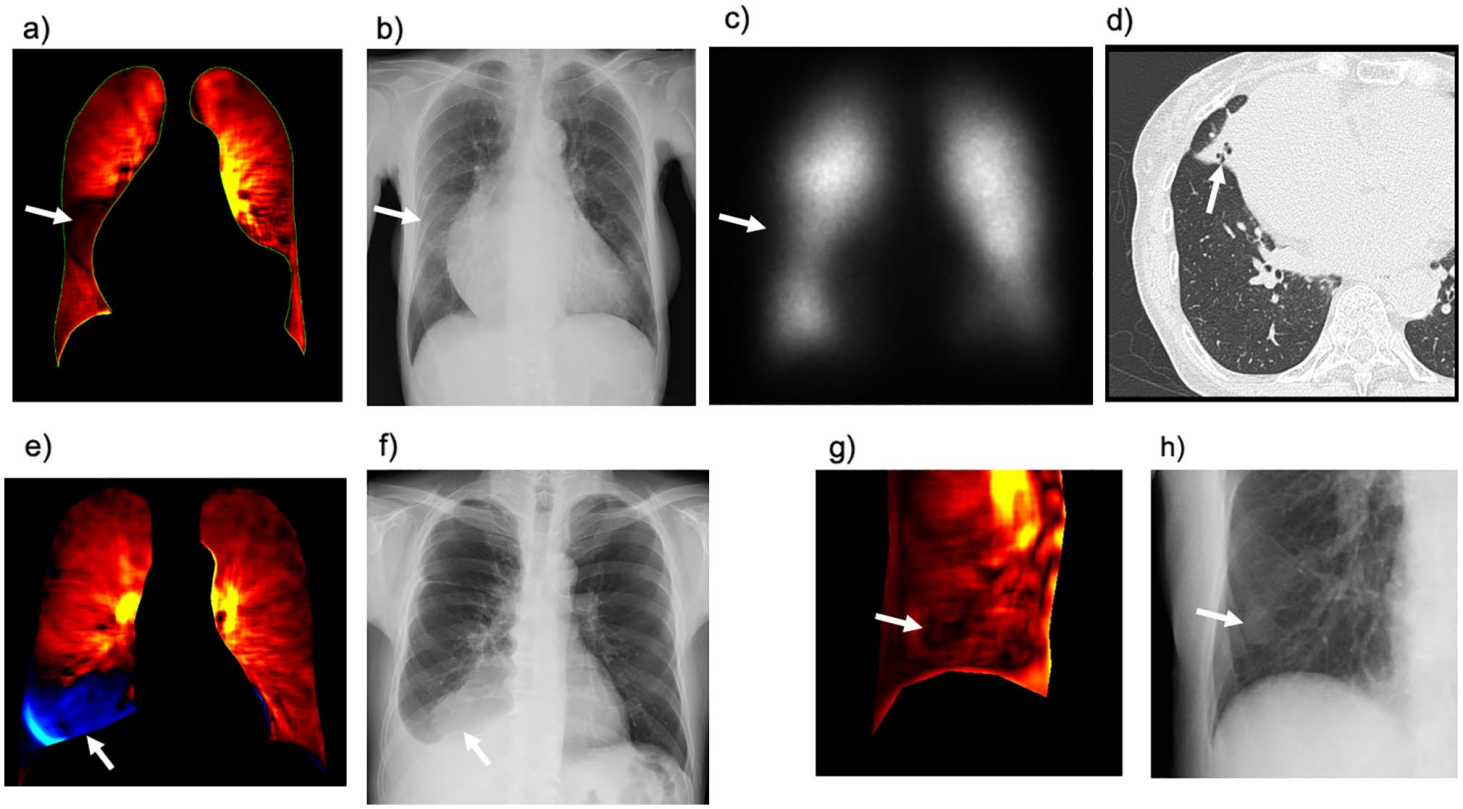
Typical pseudo-lesions of perfusion images of dynamic chest radiography (DCR): signal defects due to pulmonary or extrapulmonary lesions. Perfusion images of DCR (a), chest radiograph (b), anterior planar perfusion scintigraphy (c), and chest computed tomography (CT) (d) in a patient with atelectasis of the right middle lobe. DCR perfusion image (e) and chest radiograph (f) of a patient with right pleural effusion. DCR perfusion image (g) and chest radiograph (h) of the nipple shadow
